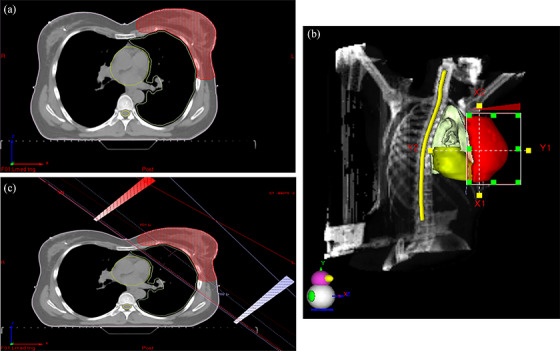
(a) Axial CT slice and (b) digitally reconstructed radiograph (DRR) showing the delineation of the bilateral lung lobes, planning target volume (PTV), and adjacent organs at risk. (c) Treatment fields encompassing medial and lateral tangents.
